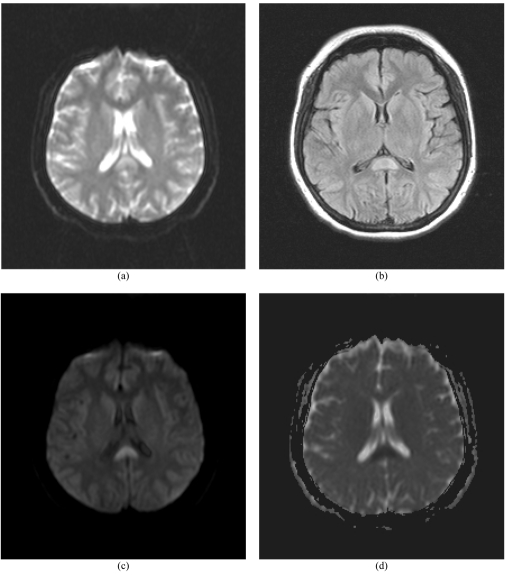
MRI imaging of the brain on admission with (a) axial T2-weighted, (b) axial FLAIR, (c) axial DWI and (d) corresponding ADC map. An ovoid lesion is seen at the centre of the splenium of the corpus callosum, hyperintense on T2 and FLAIR with markedly restricted diffusion and decreased ADC values.
